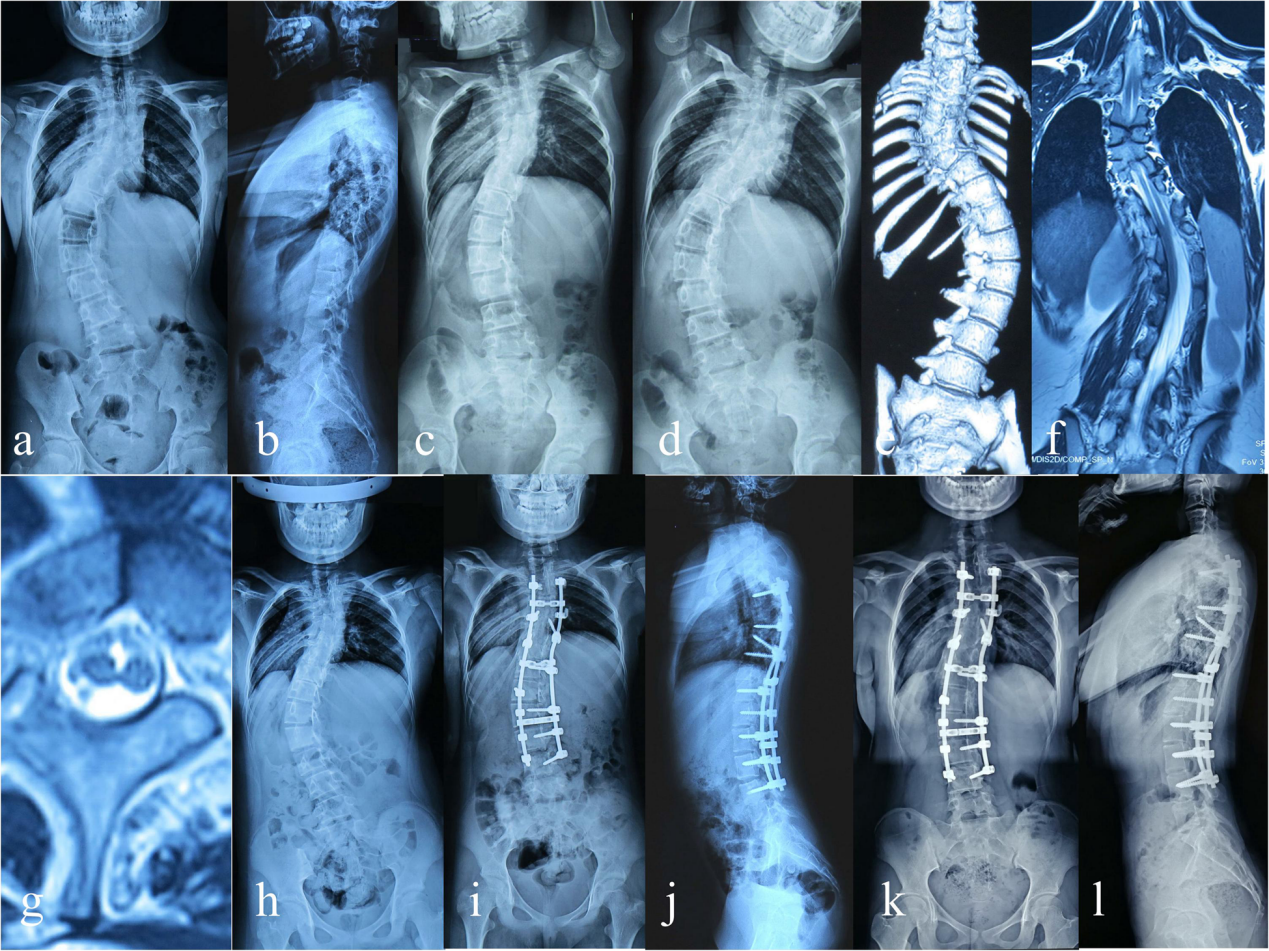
A patient with RCS accompanied by SCM. a-b Preoperative radiographs show that coronal Cobb angle was 62°.c-d Preoperative bending radiograph of the convex side shows that Cobb angle was 45.6° and flexibility was 26.45%.e Preoperative CT indicates mixed defects including failure of segmentation and formation. f-g Preoperative MRI indicates SCM type 2. h After heavy halo-femoral traction, the coronal Cobb angle was reduced to 38.2°.i-j After posterior-only surgical correction, postoperative radiographs show that coronal Cobb angle was 21.4°, and correction rate was 65.48%. k-l postoperative radiographs at 36 months after surgery show that coronal Cobb angle was 22.4°, and no signs of neurological impairment were found at the final follow-up stage
